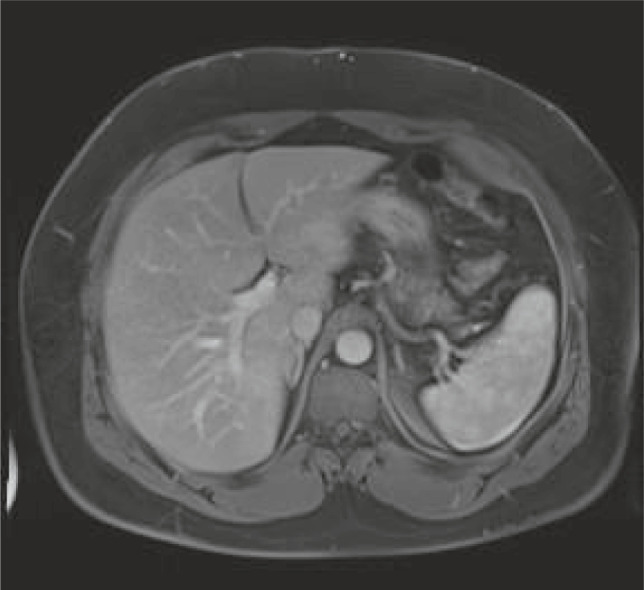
MRI findings 4 months after treatment showing complete resolution of lesions
